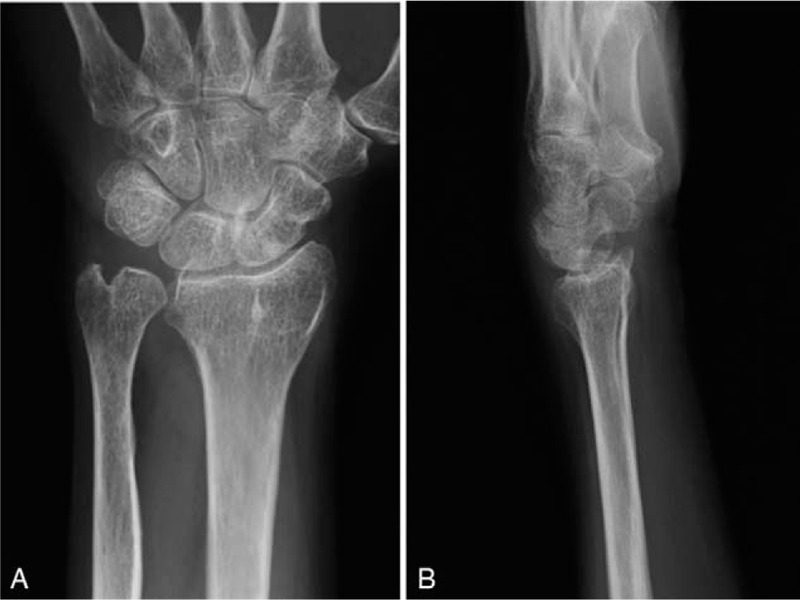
Radiographs of the left wrist at the time of presentation. Anteroposterior (A) and lateral (B) views. Although diastasis of the DRUJ is not seen on the anteroposterior view, there is slight volar displacement of the ulnar head in relation to the distal radius and carpal bones on the lateral view.
